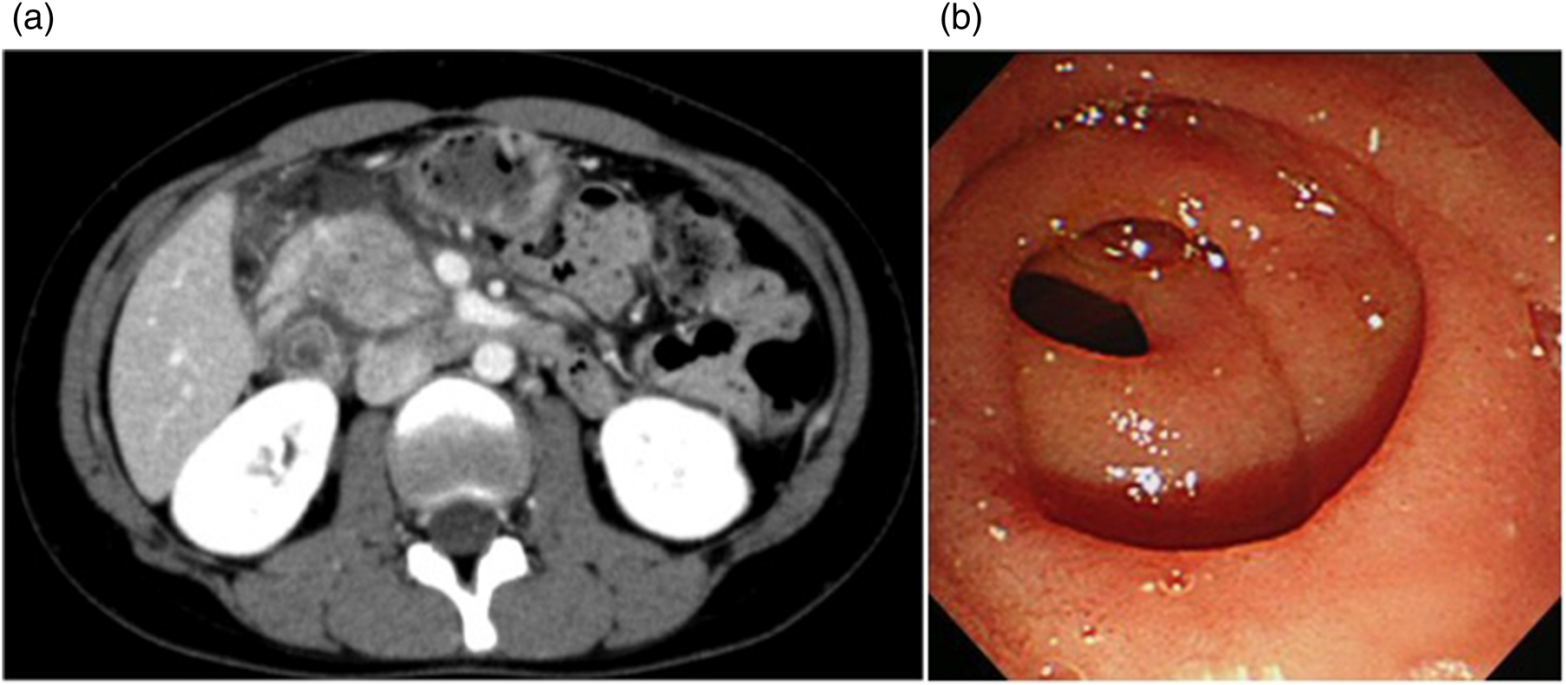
(a) Abdominal computed tomography showed acute pancreatitis localized to the annular pancreas. (b) Endoscopy revealed ring‐shaped stenosis in the descending part of the duodenum.
